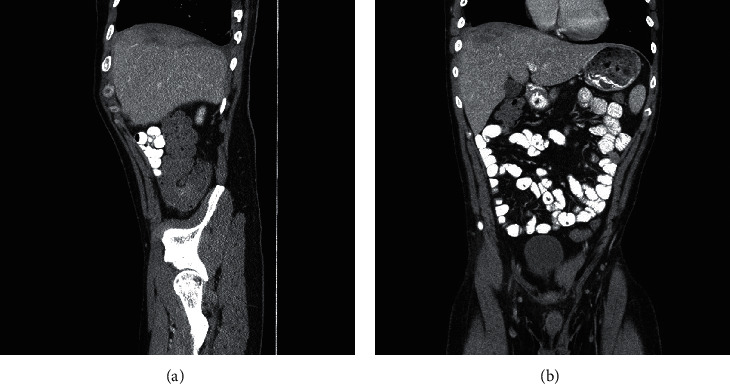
CT chest/abdomen/pelvis with contrast, sagittal view (a) and coronal view (b), 57 days after hospital admission, showing resolution of hepatic abscesses.
